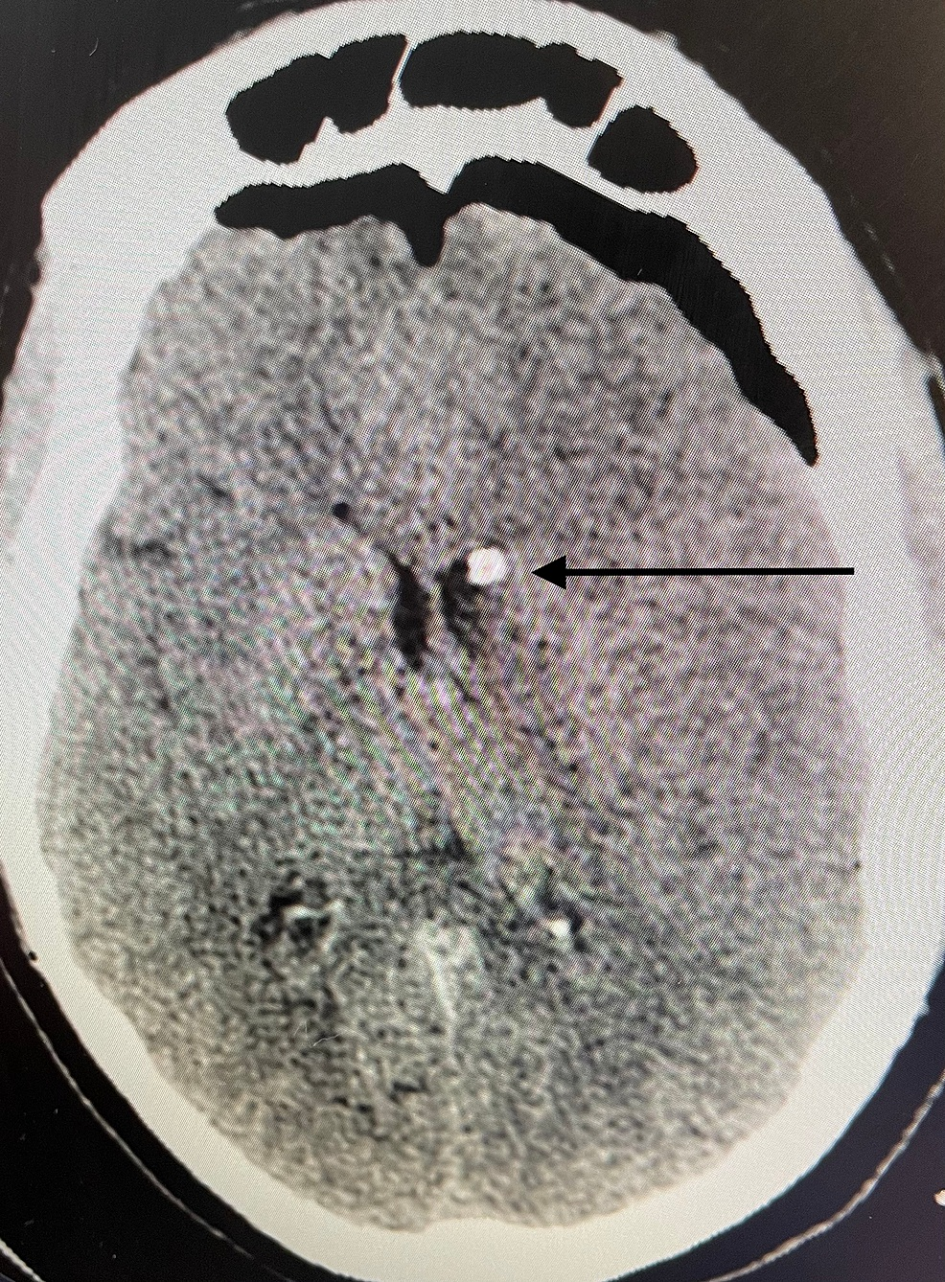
Postoperative head CT demonstrating complete resolution of left caudate nucleus intracerebral hemorrhage and left lateral ventricle intraventricular hemorrhage (arrow) CT: computed tomography
